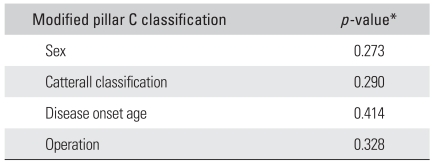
Relationship between the Modified Lateral Pillar C Classification and the Demographic and Radiographic Data.
